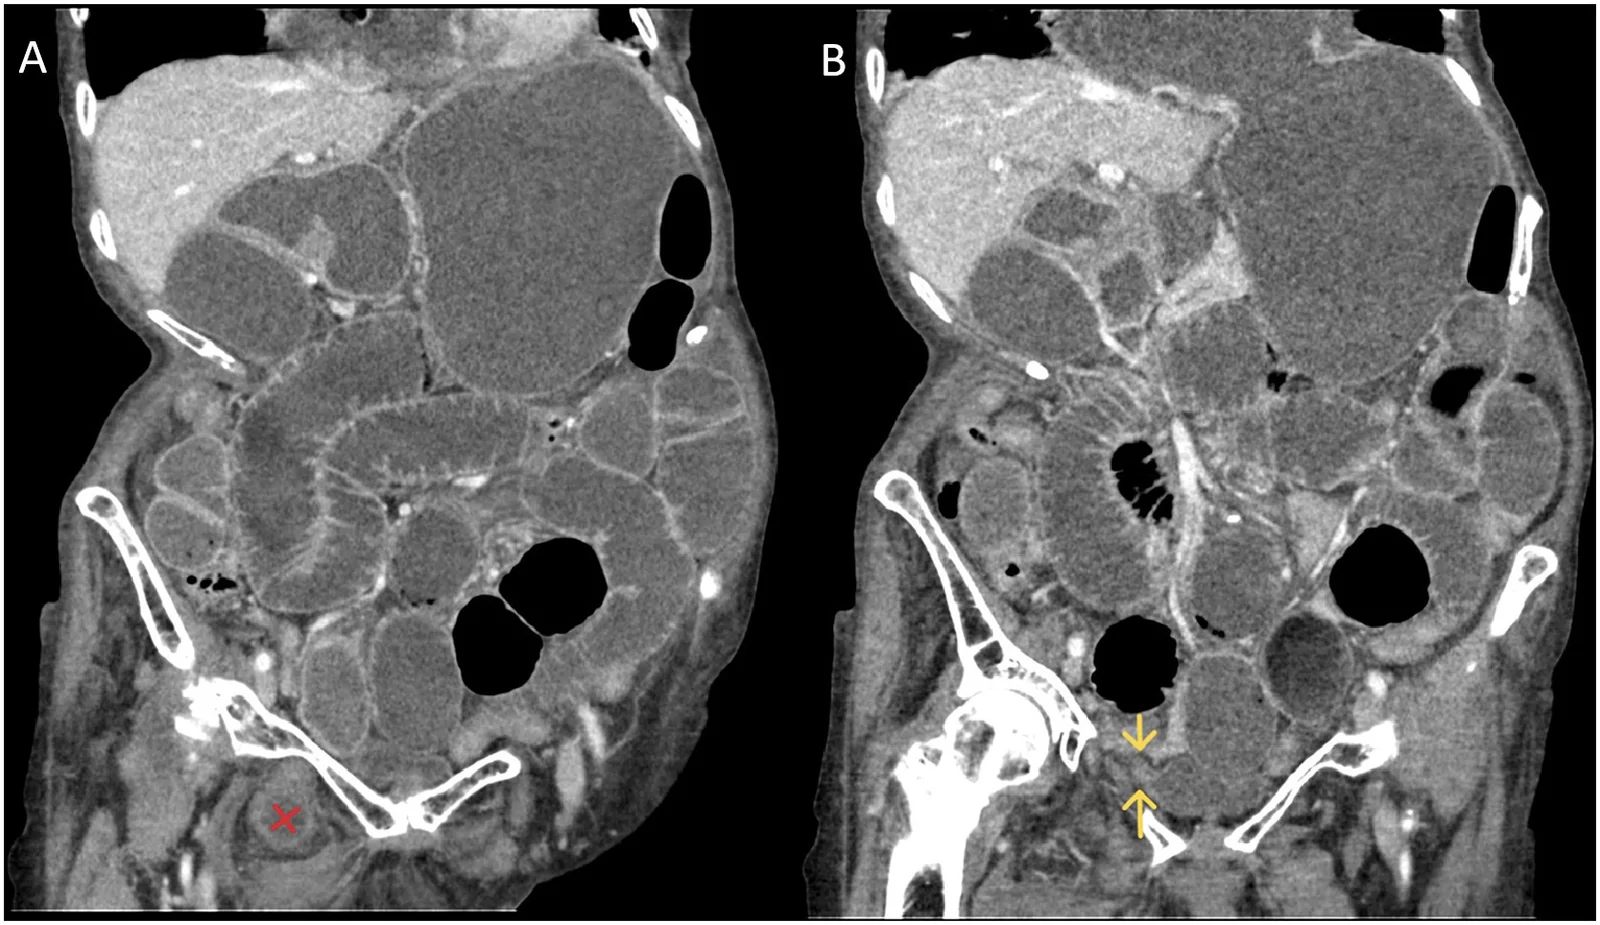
Sequential coronal images from contrast-enhanced CT of the abdomen and pelvis demonstrate: (A) diffusely dilated stomach and small bowel and herniated loop (red X); (B) transition point at the hernia neck (yellow arrows).
